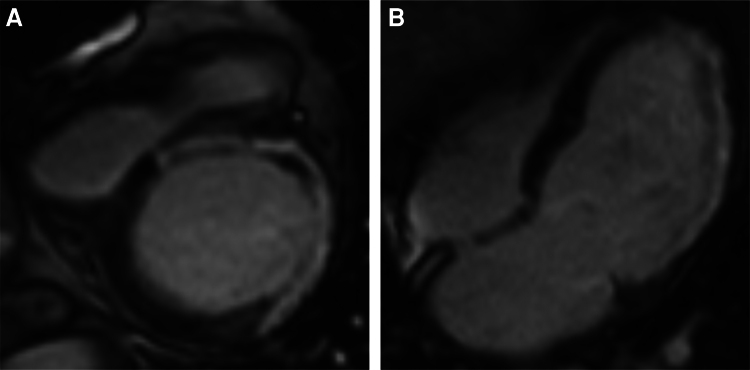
Cardiac magnetic resonance imaging showed delayed enhancement in the left ventricular septum, anterior wall, lateral wall, and apex (A and B)
